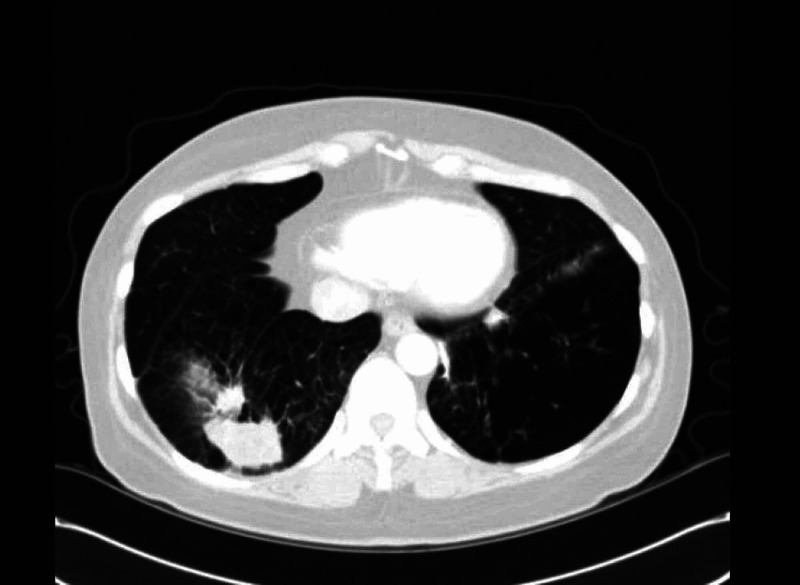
CT of the chest three months later There is persistent right lower lobe lung mass with small mass slightly superior, which has reduced in size compared to prior CT. New left-sided lung nodule was present.
